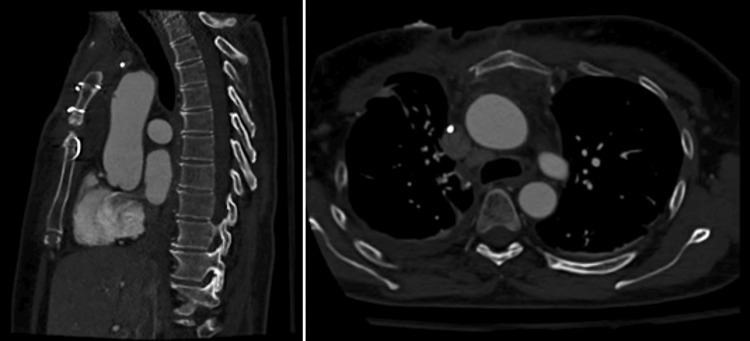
Postoperative computed tomography angiography after removal of the sternal wire
